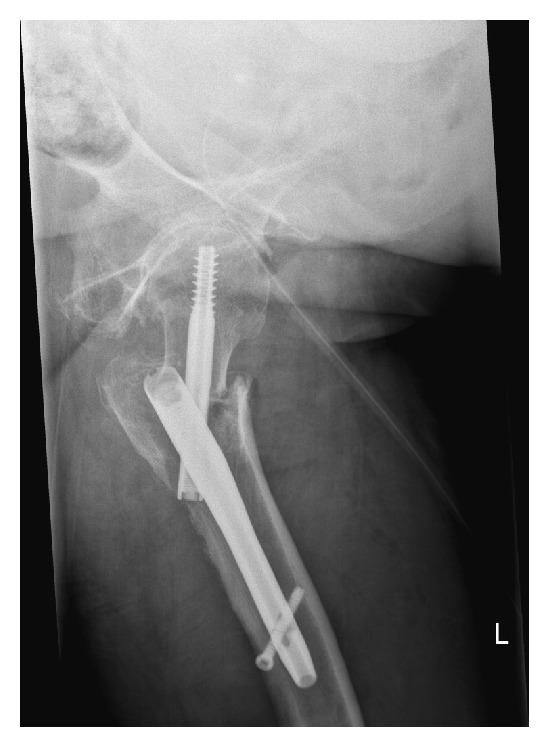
Axial postoperative X-ray showing good positioning of the intramedullary Gamma nail in the right femur.
